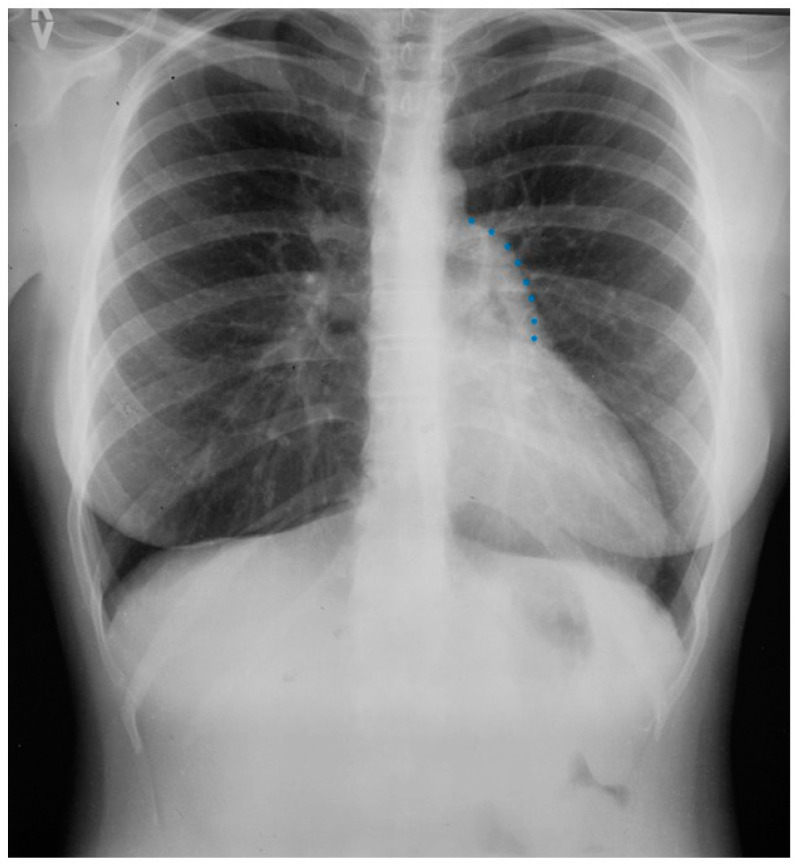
Posteroanterior chest X-ray in congenital complete absence of the left portion of the parietal pericardium: A 30-year-old female was referred to a cardiologist because of an unusual chest X-ray done as part of an executive physical. She was asymptomatic and her vital signs and heart sounds were normal and there was no heart murmur or arrhythmia. The image is well centered, there is a good inspiration and the C-T ratio is <0.5. The heart is shifted into the left chest and the right heart border is obscured because it overlies the dorsal vertebral bodies. The pulmonary artery segment (outlined with blue dots) is enlarged but the pulmonary vascularity is normal, making left to right shunting unlikely. Echocardiography and Cardiac MRI confirmed she had CCALPPP. She was reassured. No treatment was necessary.
